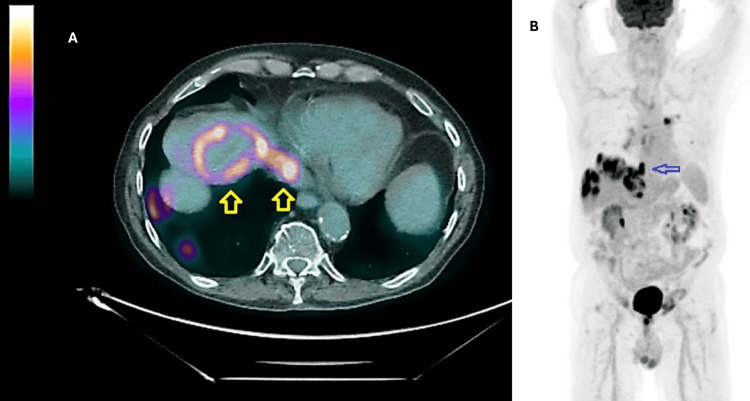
Positron-emission tomography (PET) scans demonstrating intravascular tumor extension A) A fused, axial PET CT scan of the abdomen showing intense 18-F fluorodeoxy glucose (FDG) uptake along a hepatic tumor lesion and extending along the middle hepatic vein into the inferior vena cava (arrows). B) A coronal PET scan demonstrated an FDG-intense band extending from liver lesion into the inferior vena cava toward the right side of the heart (arrow).
